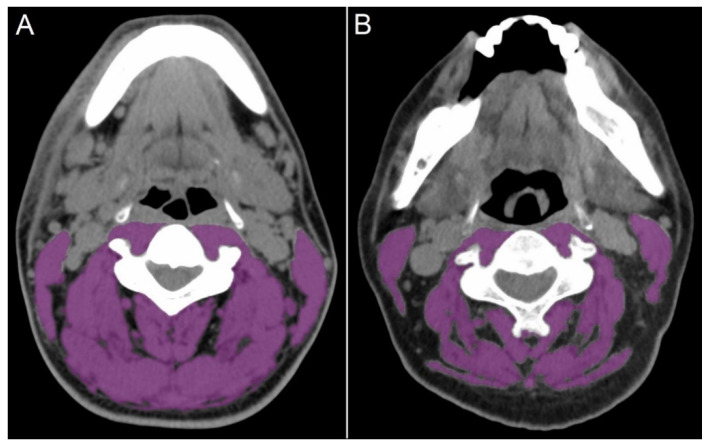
Skeletal muscle area regions in computed tomography images at the third cervical vertebral level in patients without (A) and with (B) sarcopenia.
